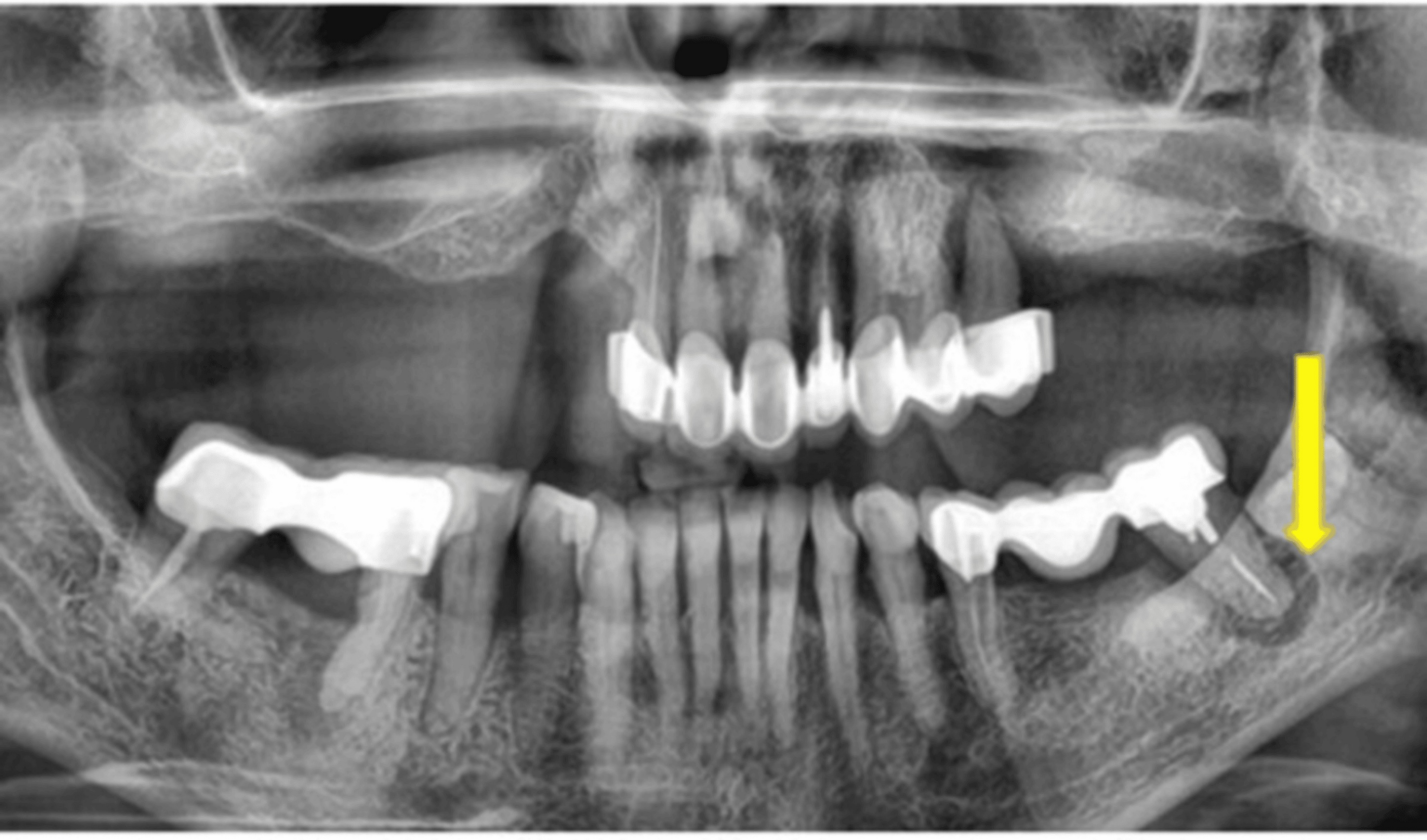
Giant periapical abscess (arrow); the tooth is only held by the bridge Image Credit: Dr. Zoltán Varga
